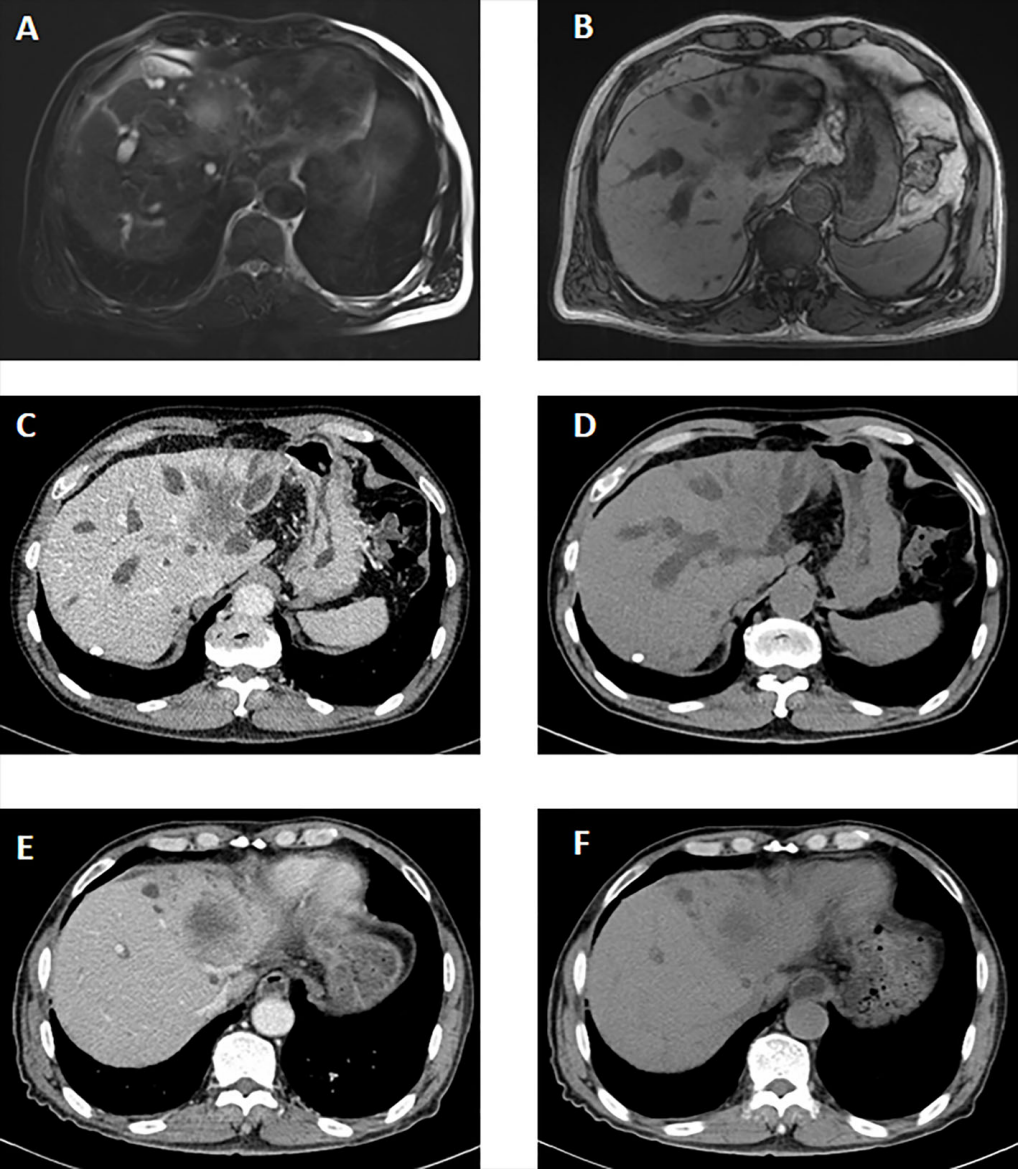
The imaging manifestations of ASC in liver. The MRI features before the therapy. (A, B) It showed a long T2 signal mass with heterogeneous enhancement in arterial phase and delayed enhancement in portal phase in the hilar and left lobe of liver. A slightly long T1 signal mass with an unclear boundary was seen. The CT features before the therapy. (C, D) A slightly low-density mass was seen in the hilum and left lobe of liver, about 6.1*3.9 cm. On contrast-enhanced scan, the lesions were slightly inhomogeneous and mainly marginal. The CT features after percutaneous transhepatic cholangial drainage (PTCD) and targeted therapy. (E, F) Low density mass shadow was seen in the hilus hepatis and left lobe of liver with unclear boundary. The enhancement degree of lesions after enhanced scanning was lower than that before treatment.
